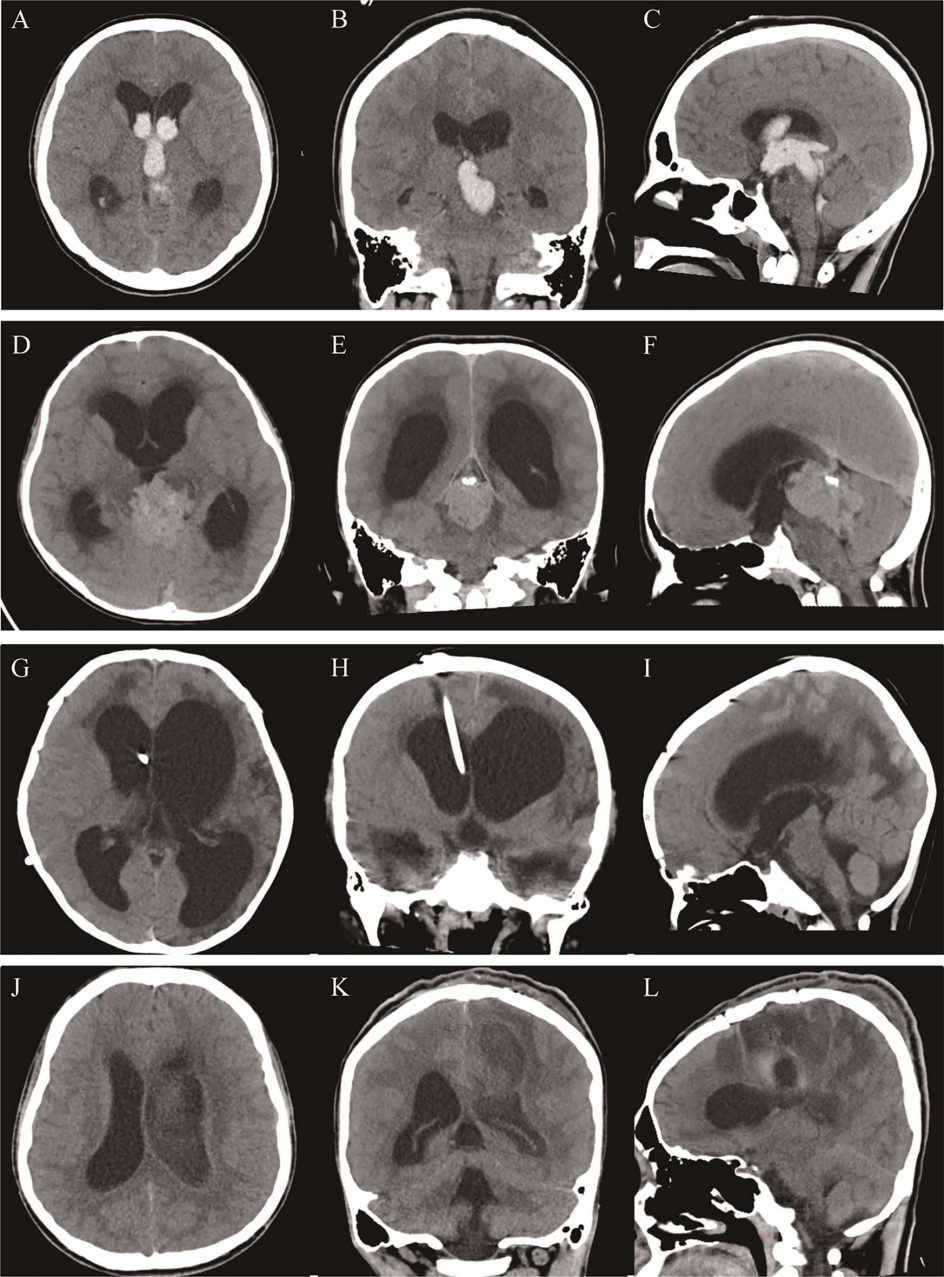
CT imaging features of various acquired hydrocephalus. Imaging of secondary hydrocephalus due to intraventricular hemorrhage: Axial (A), coronal (B), and sagittal (C), Imaging of obstructive hydrocephalus caused by brain tumor: Axial (D), coronal (E), and sagittal (F), Imaging of secondary intracranial infection after ventriculoperitoneal shunt: Axial (G), coronal (H), and sagittal (I), Imaging of brain abscess with intracranial infection: Axial (J), coronal (K), and sagittal (L).
